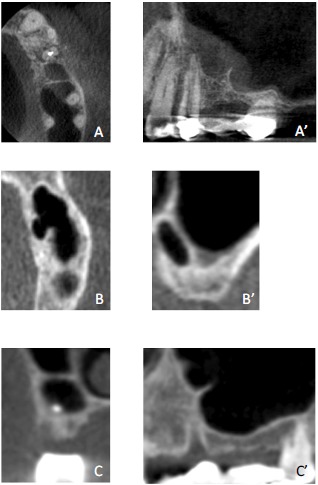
CBCT scan showing septum classification according to direction.A & A’: Class I septum in buccal lingual direction, A: Transverse Plane. A’: Sagittal PlaneB &B’: Class II septum in mesial distal direction, B: Transverse Plane. B’: Coronal PlaneC & C’: Class III septum in horizontal direction, C: Coronal Plan. C’: Sagittal Plane.
